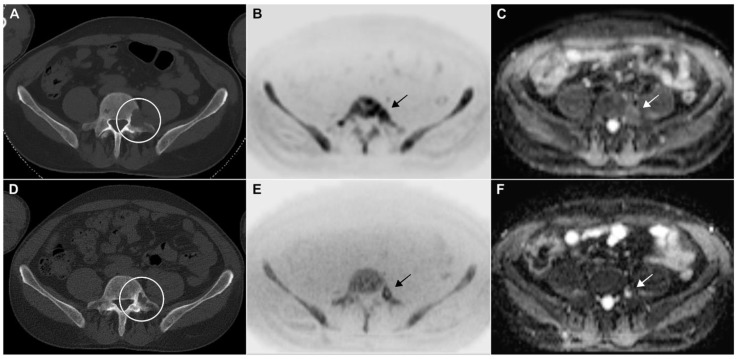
Baseline CT in a 48-year-old man with newly diagnosed multiple myeloma (IgG lambda, R-ISS I) depicted a large osteolysis in lumbar vertebra 5 ((A): circle) with active tumor signal in diffusion-weighted MRI ((B): inverted gray scale image with b-value of 2000 s/mm^2^; (C): ADC map; arrows). After six cycles of E-KRd, the lesion was significantly smaller, exhibiting considerable remineralization ((D): circle). Hypocellular matrix and high ADC signal in MRI ((E,F): arrows).
